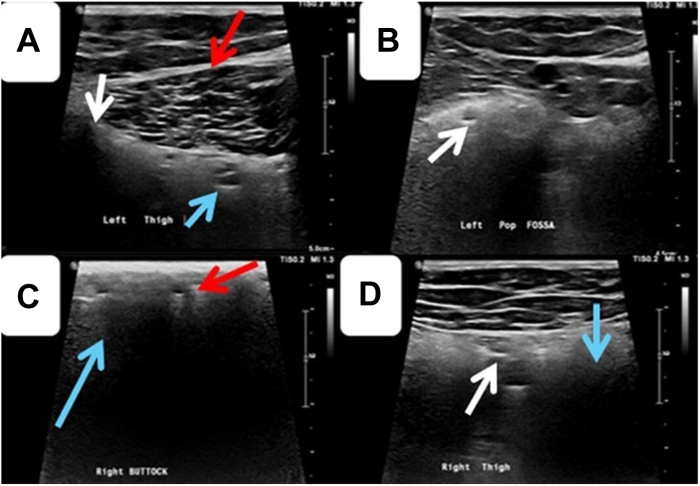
B-Mode Ultrasound of the Bilateral Lower Extremities Demonstrating Migrated Injectable Material (A) B-mode ultrasound of the left thigh demonstrates preserved muscle belly architecture (red arrow) with involvement of the deep fascial plane, where heterogeneous hyperechoic material containing multiple cystic spaces is seen (blue arrow). Prominent posterior acoustic shadowing surrounds the muscle and deep fascia, consistent with foreign material deposition (white arrow). (B) Ultrasound of the left popliteal fossa shows cysts (white arrow). (C) B-mode ultrasound of the right buttock reveals ill-defined hyperechoic soft tissue changes with multiple small cystic areas (red arrow) and a characteristic “snowstorm” artifact with posterior shadowing (blue arrow), suggestive of migrated injectable material. (D) Ultrasound of the right thigh demonstrates more extensive cystic changes (white arrow), marked hyperechogenicity, loss of normal tissue planes, and prominent posterior acoustic shadowing (blue arrow), consistent with migrated injectable material associated with a chronic inflammatory granulomatous reaction.
